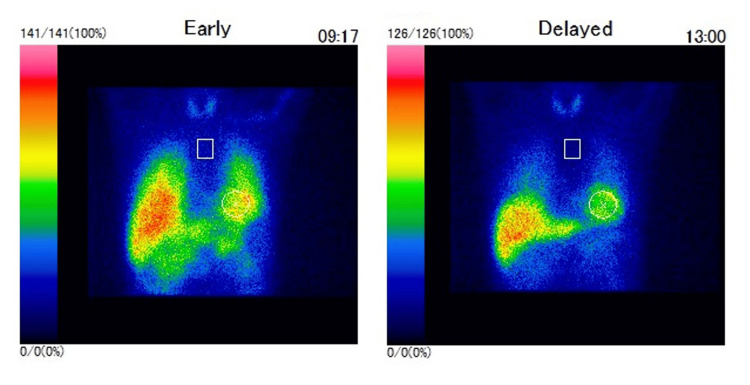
Results of the 123I-MIBG cardiac scintigraphy The^ 123^I-MIBG cardiac scintigraphy demonstrated a preserved accumulation of nuclides in the heart. The heart-to-mediastinum ratio (H/M ratio) mean values were 3.68 for the early (15-minute) acquisition and 4.55 for the late (240-minute) acquisition.
